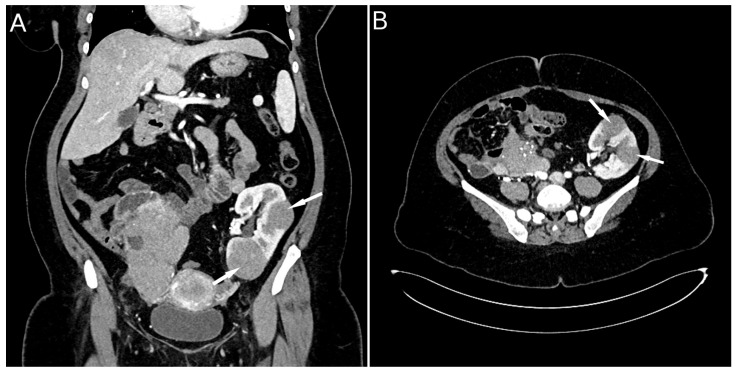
CT scan image: lesions in the transplanted kidney (A) and post-transplant lymphoma in a kidney allograft (B) (indicated by arrows).
